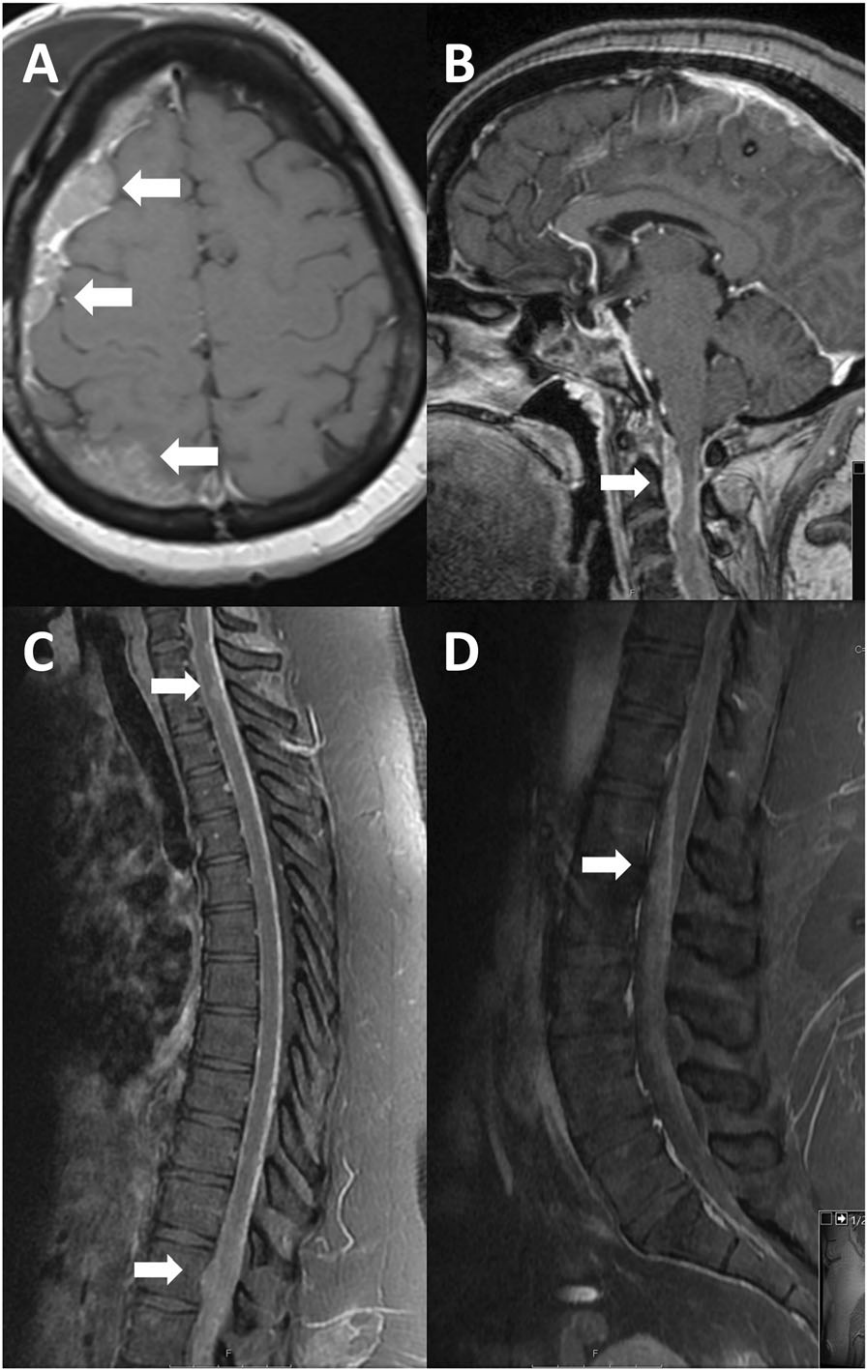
Radiographic images of leptomeningeal progression. Magnetic resonance images of the brain and spine are presented in the axial and sagittal planes, respectively. The tumor metastasized to the subdural space along on the right convexity (a) and the ventral cervical spine (b). Multiple areas of enhancement were present in the thoracic spine (c) and along the nerve roots of the cauda equina (d)
